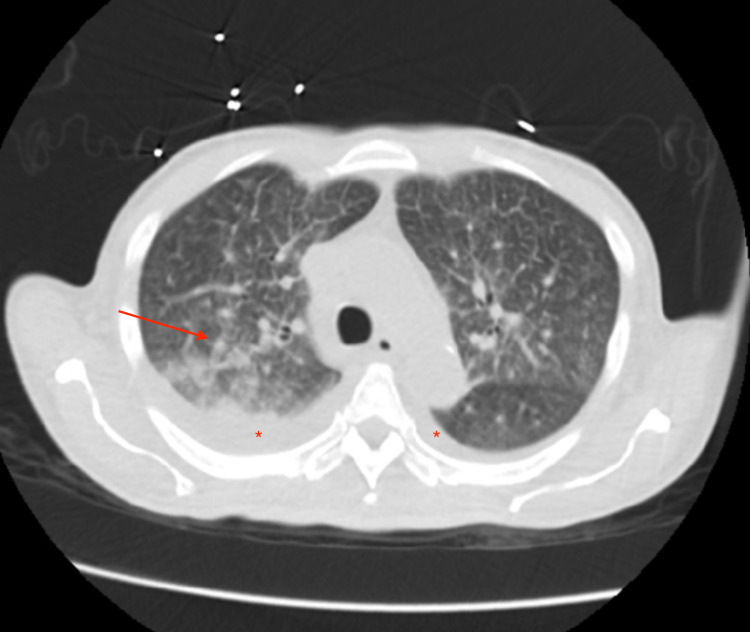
CT scan of chest arrow displaying ground-glass consolidation, asterisks highlighting bilateral effusions
